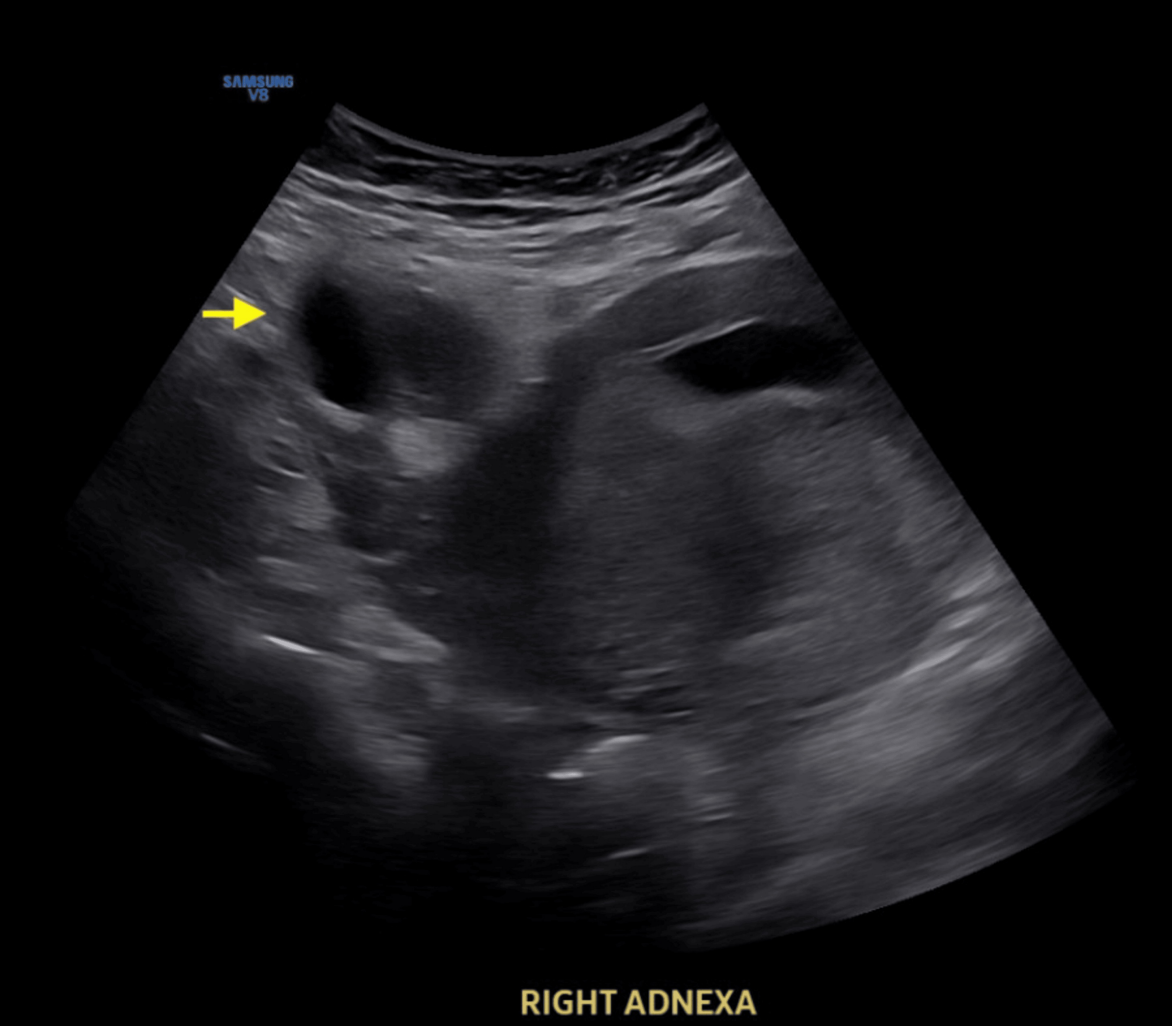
Transabdominal ultrasound of a right anechoic cyst (arrow) measuring 4.9 x 3.8 x 6.1 cm adjacent to the right ovary.
